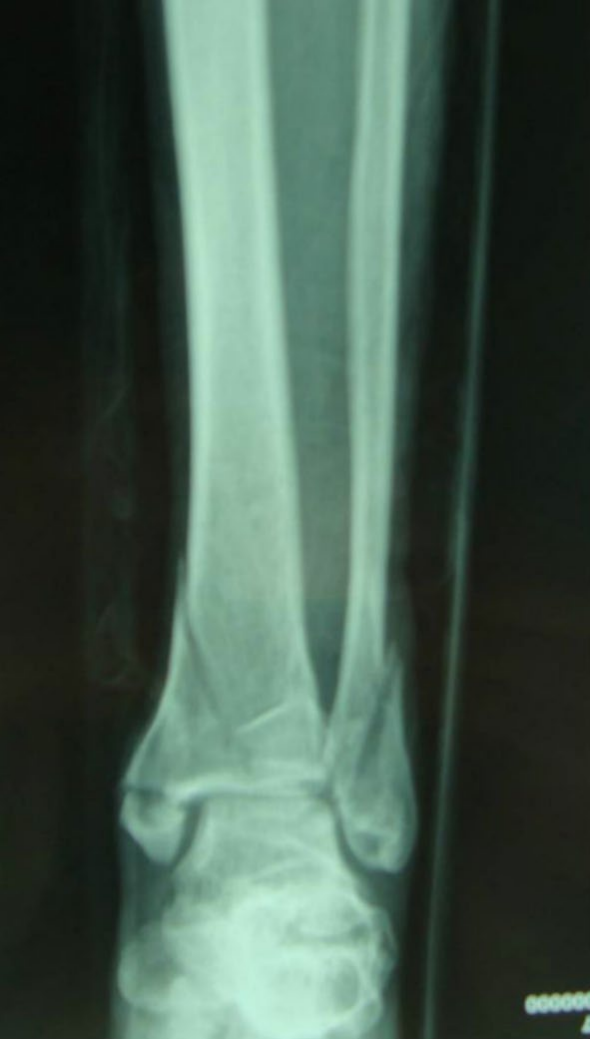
A 46 male patient had right closed pilon fracture caused by trauma (AO C1) preoperative X-ray result of right ankle joint
